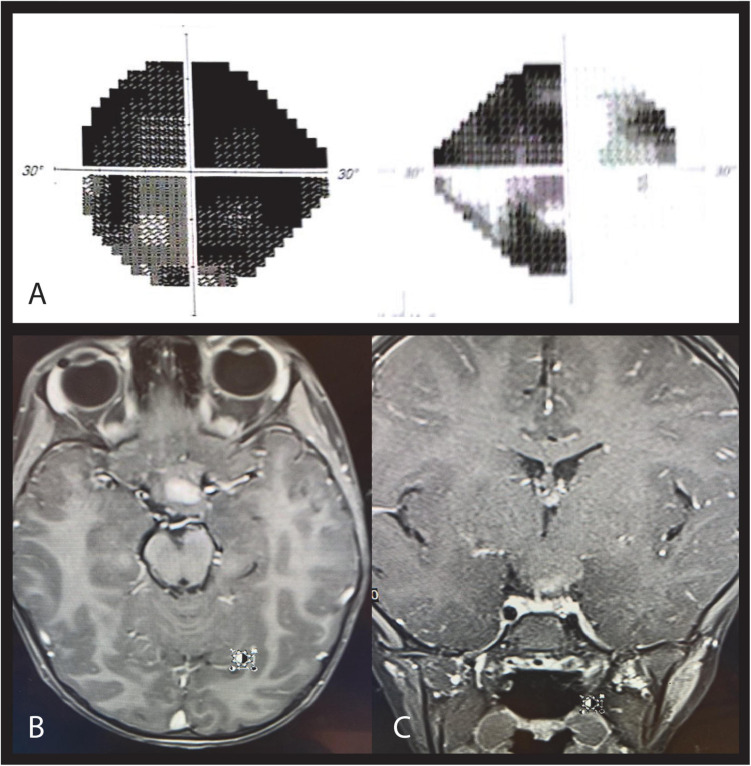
Case 5. Automated perimetry demonstrating a nasal defect respecting the vertical meridian in the right eye and a severely damaged field on the left (A). MRI T1 post contrast axial (B) and coronal (C) scans showing a large chiasmatic hypothalamic enhancing lesion extending to the left optic tract and the left pre-chiasmatic optic nerve.
